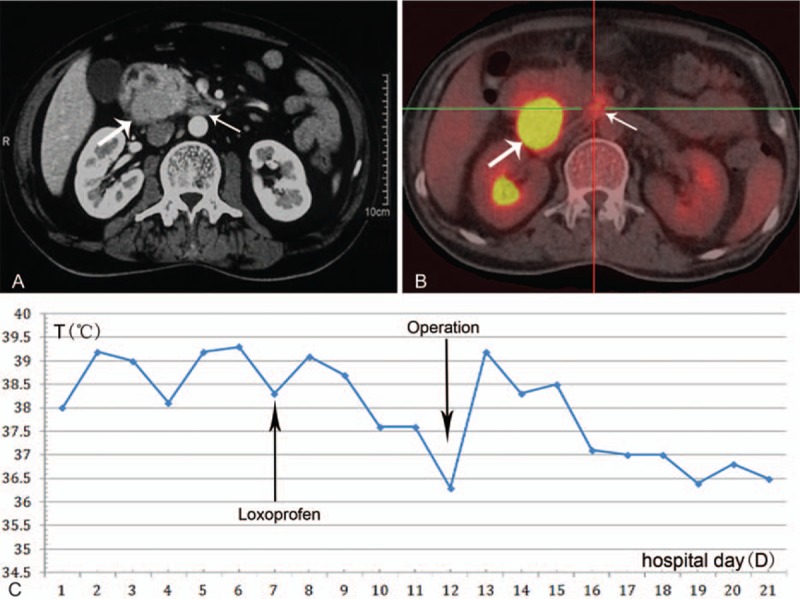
(A) Abdominal CT scan revealed a tumor between the duodenum and the pancreatic head (bold arrow) and enlarged retroperitoneal lymph nodes (thin arrow). (B) PET-CT revealed the mass (bold arrow) and enlarged lymph nodes (thin arrow) to have significantly elevated standard uptake value (SUV). (C) Temperature decreased to below 37.6 °C for 2 days before the surgery due to the therapy with loxoprofen, and complete resolution occurred on postoperative day 4. CT = computed tomography, PET-CT = positron emission tomography-computed tomography.
